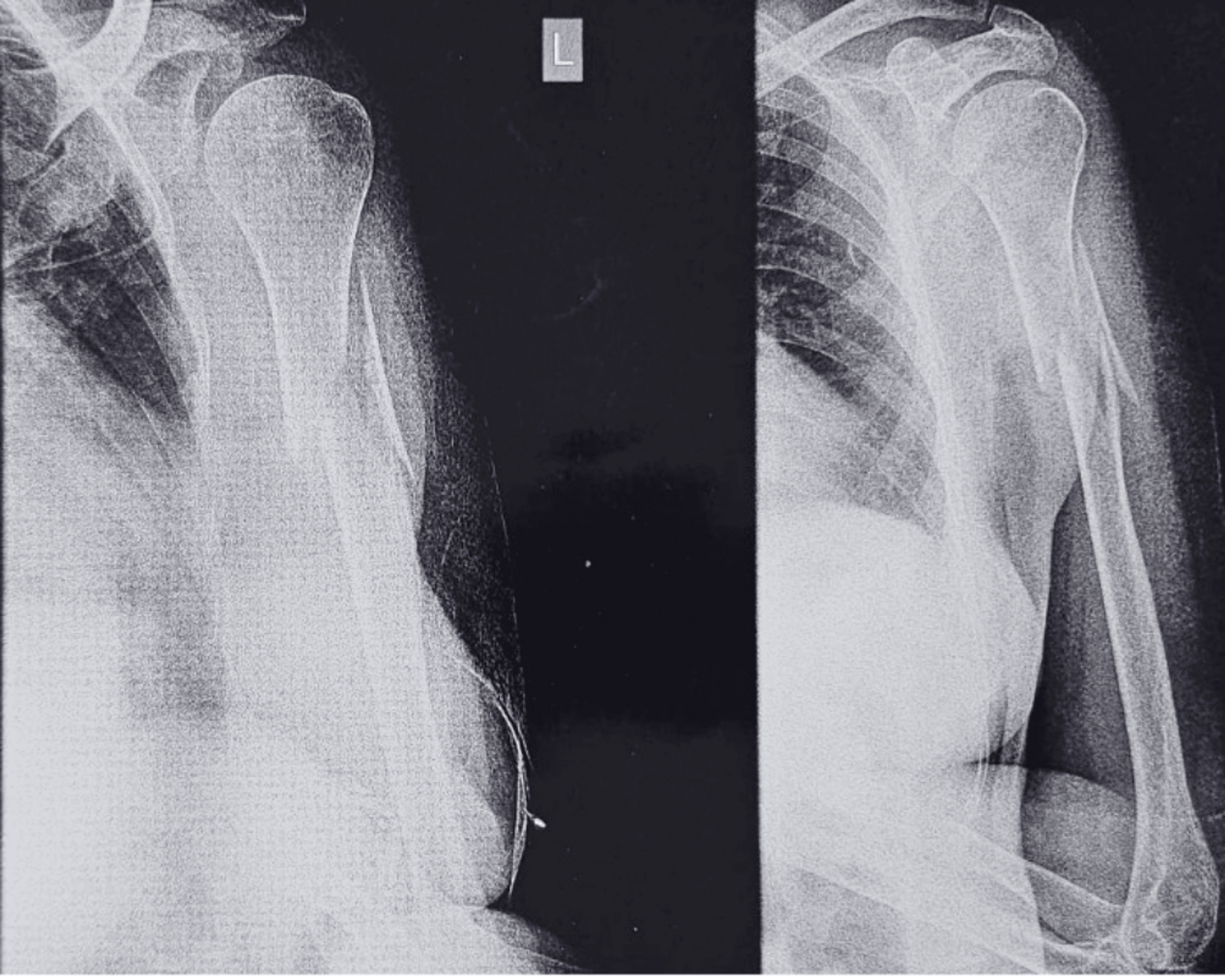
Pre-operative X-ray showing left humerus proximal 1/3 shaft fracture.
